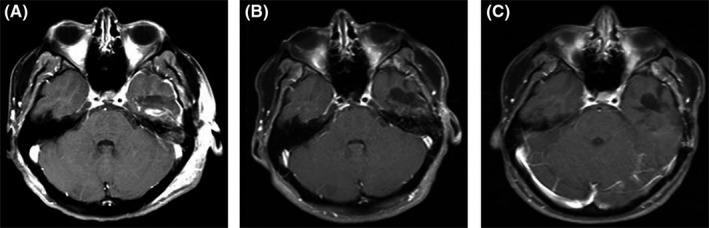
Postoperative T1 postcontrast MRI. A, 24‐hour postoperative MRI reveals gross total resection with a signal in the posterior resection bed consistent with blood products and proteinaceous fluid. B, 3‐mo and C, 6‐mo postoperative MRIs show T1 hypointensity in left temporal lobe without areas of residual or new enhancement
